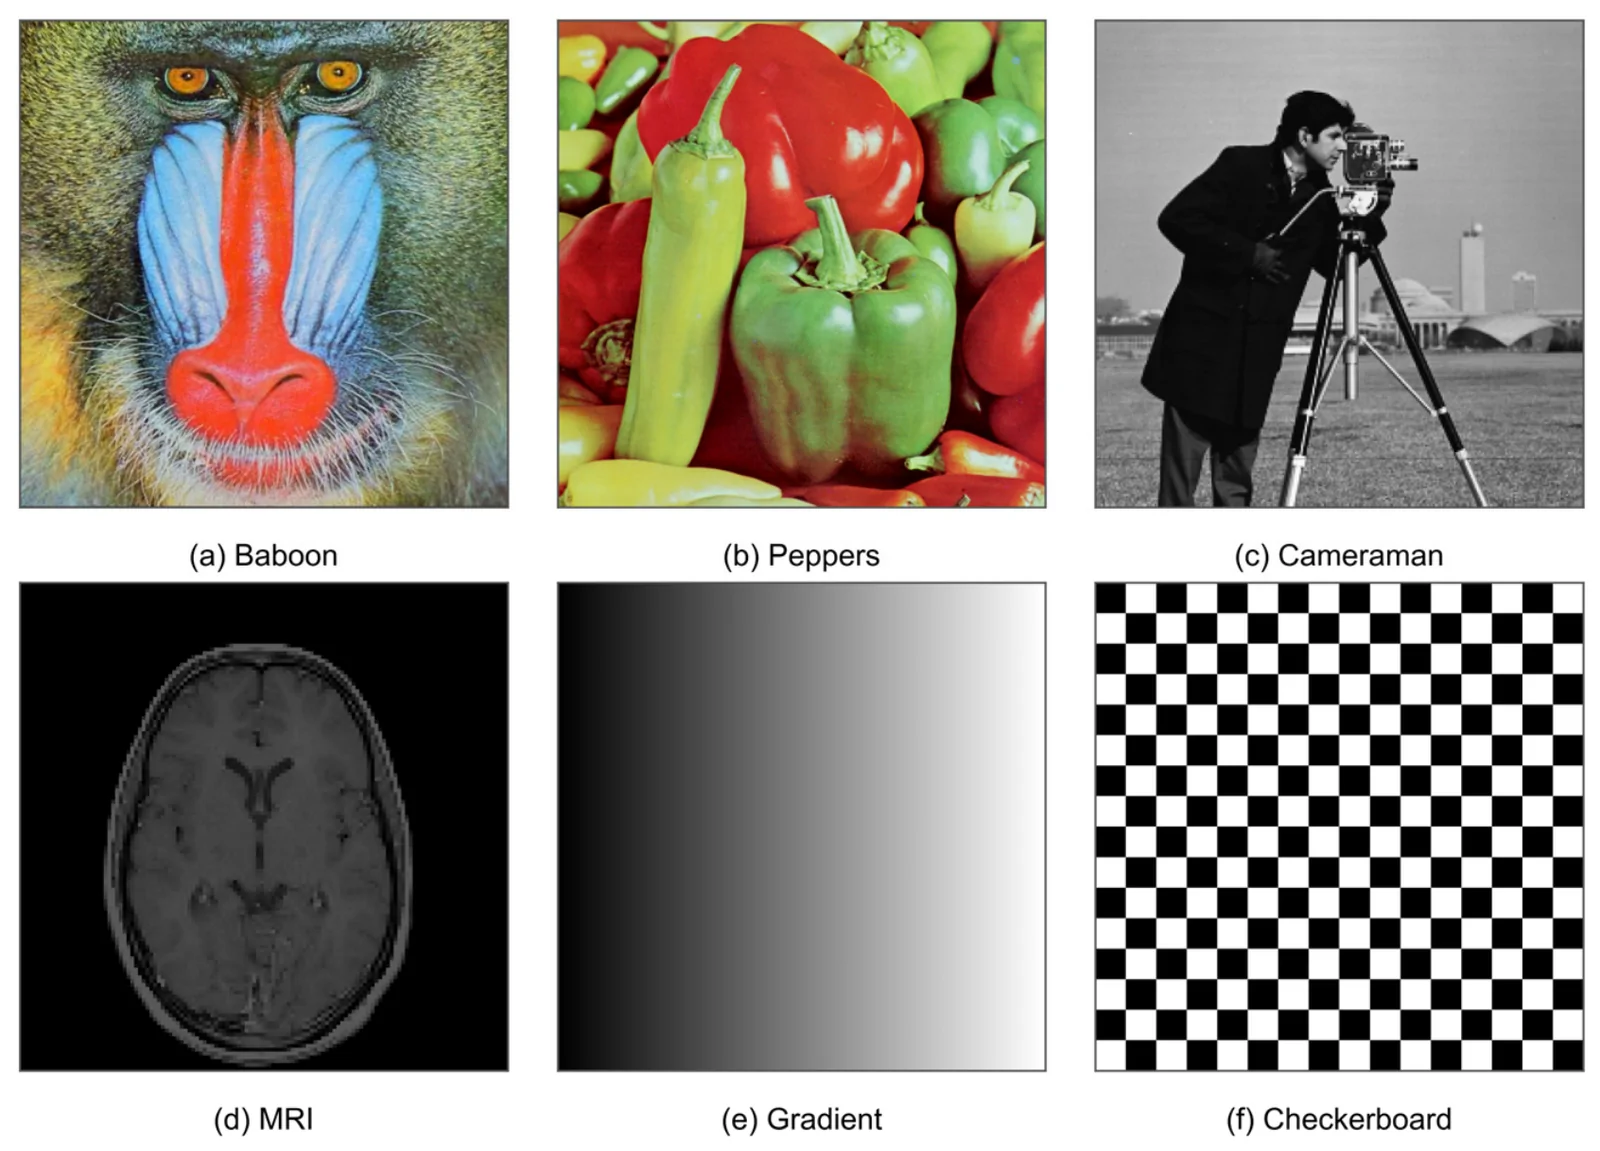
Test inputs used in the multi-image and multi-key evaluation: (a) Baboon; (b) Peppers; (c) Cameraman; (d) MRI; (e) Gradient; and (f) Checkerboard.
